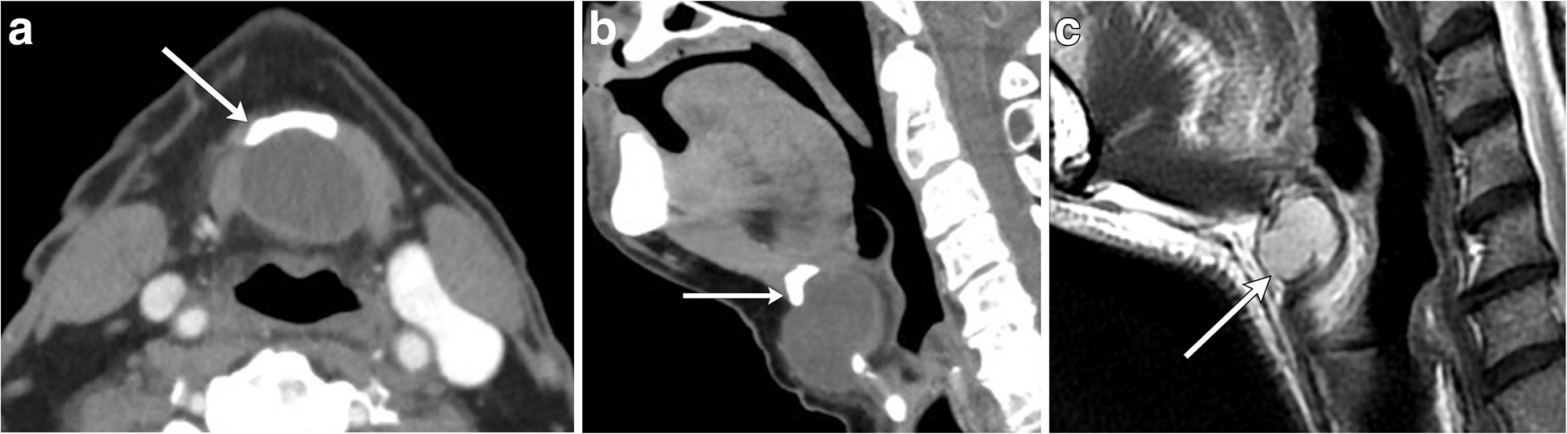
Midline thyroglossal duct cysts. Axial (a) and sagittal (b) contrast-enhanced CT images of the neck demonstrate the close relationship of a thyroglossal duct cyst (non-enhancing cystic structure within the midline neck) with the hyoid bone (arrows). c Sagittal T2-weighted MR image of a different patient reveals a fluid signal lesion extending posterior to the hyoid bone (arrow)
